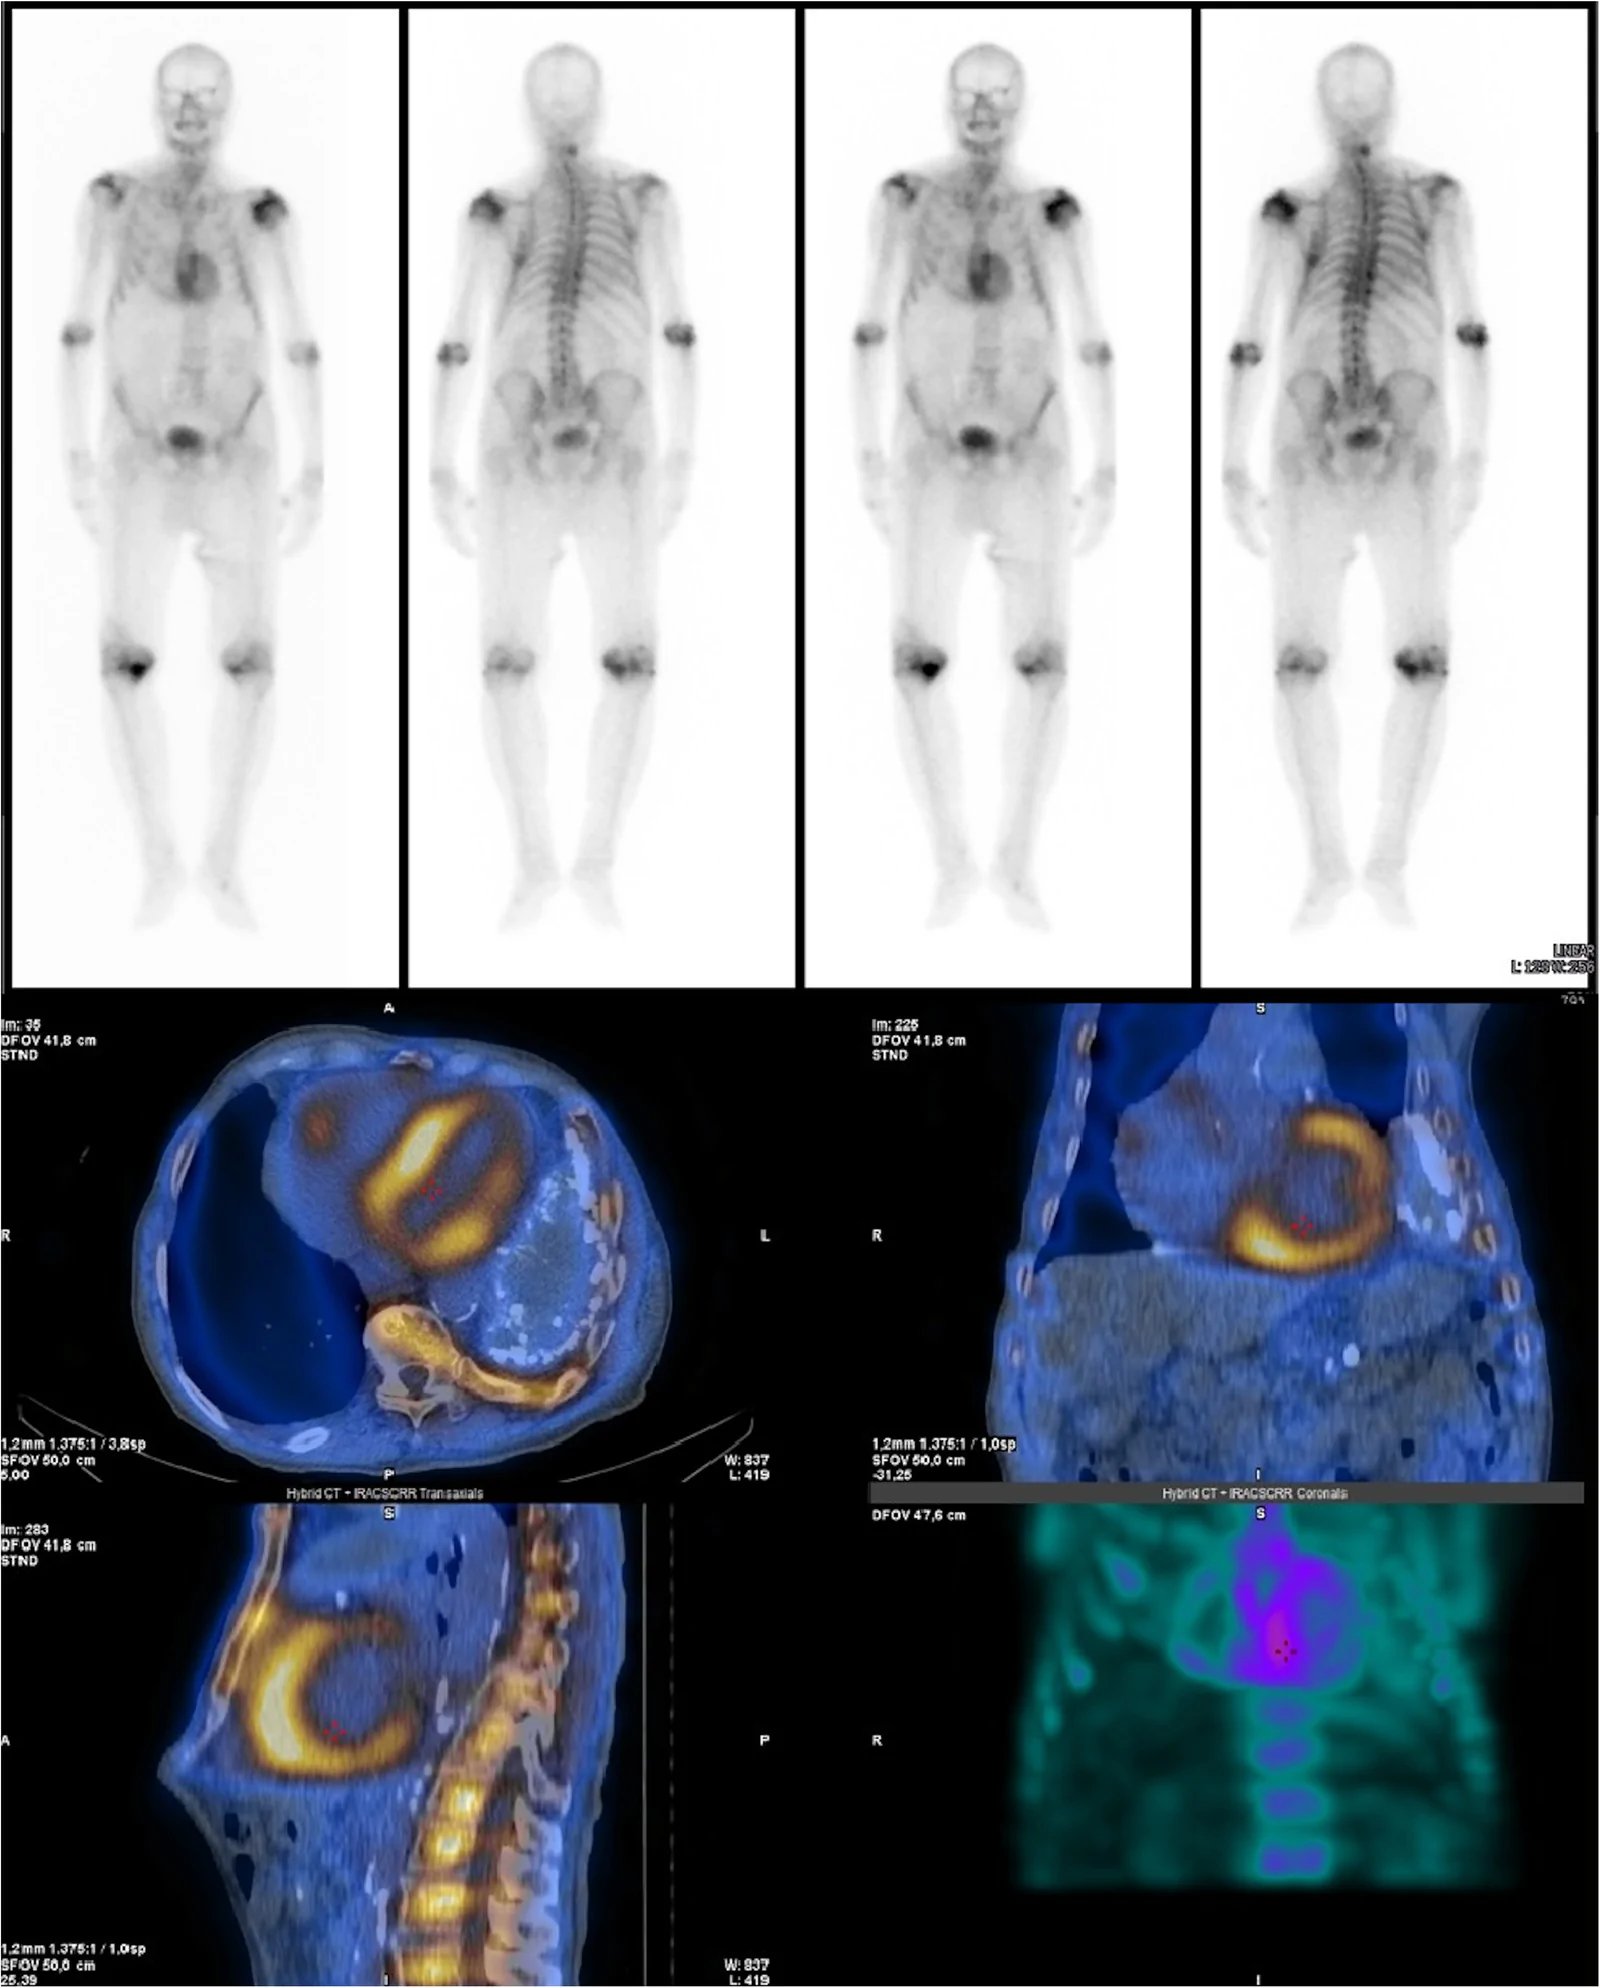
Tc-99m Pyrophosphate Scintigraphy Showing Perugini Grade 3 Myocardial Uptake (Top) Whole-body planar bone scintigraphy demonstrates intense and diffuse myocardial tracer uptake exceeding bone uptake, consistent with Perugini grade 3 myocardial uptake. (Bottom) Hybrid SPECT/CT images (axial, coronal, sagittal, and maximum intensity projection) confirm diffuse myocardial tracer accumulation. SPECT/CT = single-photon emission computed tomography/computed tomography.
